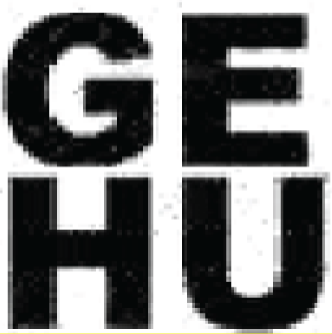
The article explains how the watermarked image can be damaged in numerous ways and the recovered watermark will still be legible. Yet, there is a trade-off to consider, since the approach’s durability and the quality of the watermarked image are inversely related (Singh et al., 2022). For NIfTI pictures generated by CT scan, the suggested watermarking procedure results in a watermarked NIfTI image for safe diagnosis. Because a considerable drop in watermarked picture quality is dangerous and might result in the patient’s death, the suggested approach is evaluated against several noise attacks. The efficiency and dependability of the suggested approach for diagnostic purposes are demonstrated by the quality metrics and parameters indicating that the watermarked picture keeps excellent quality even after being attacked.
